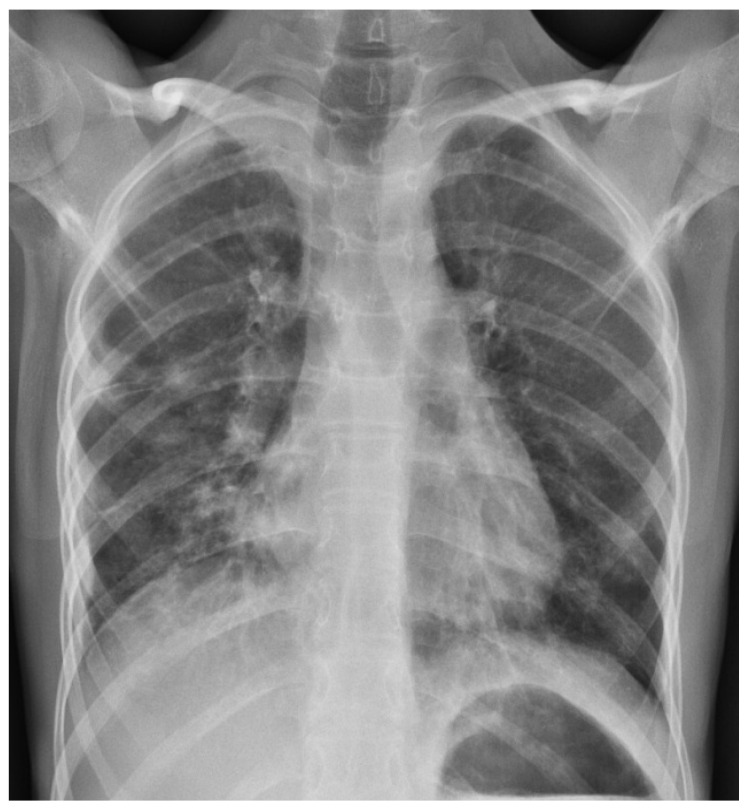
Anteroposterior (AP) chest radiograph of a 15-year-old adolescent with recurrent aspiration pneumonia. Alveolar consolidation foci are observed in the left retrocardiac area, as well as right infrahilar alveolar infiltrates, a rounded right lateral costophrenic sinus, and a sharp left lateral costophrenic sinus.
